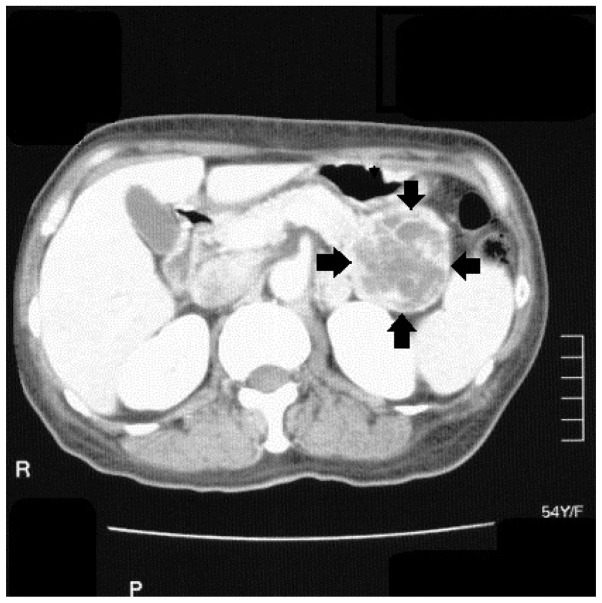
Abdominal computed tomography showing a well-encapsulated tumor located at the tail of the pancreas. (indicated by arrows).
